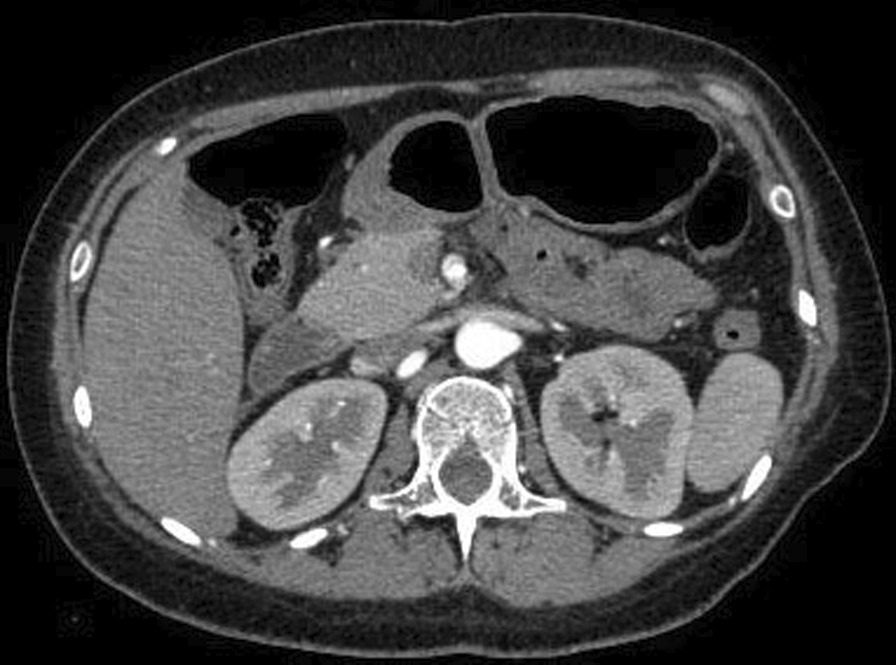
Computed tomography angiography (CTA) demonstrated a marked superior mesenteric artery dissection
